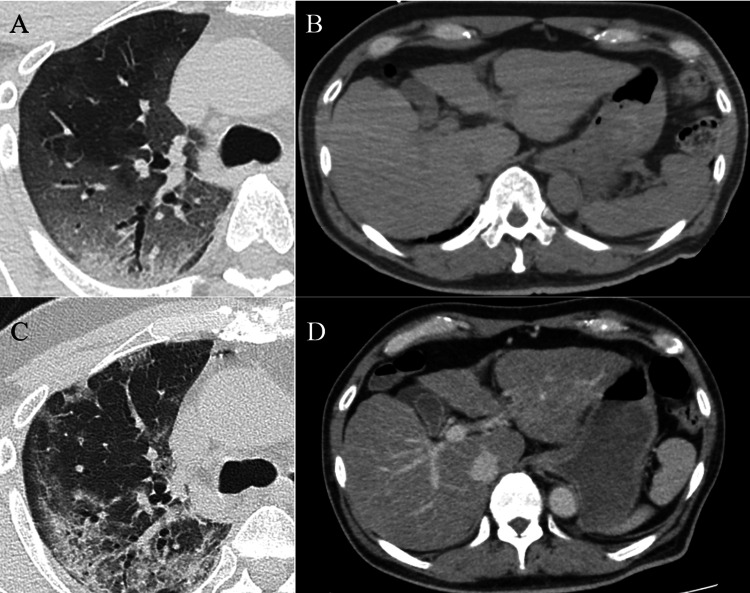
Changes in CT images throughout the clinical course. Chest and abdominal CT images at hospital admission (A, B) and intensive care unit admission (C, D). Ground-glass opacities were diminished and organization was observed (A, C). The gallbladder was edematously thickened, suggesting liver damage such as hepatitis or shock liver. No hepatic atrophy or splenomegaly was noted (B, D).
